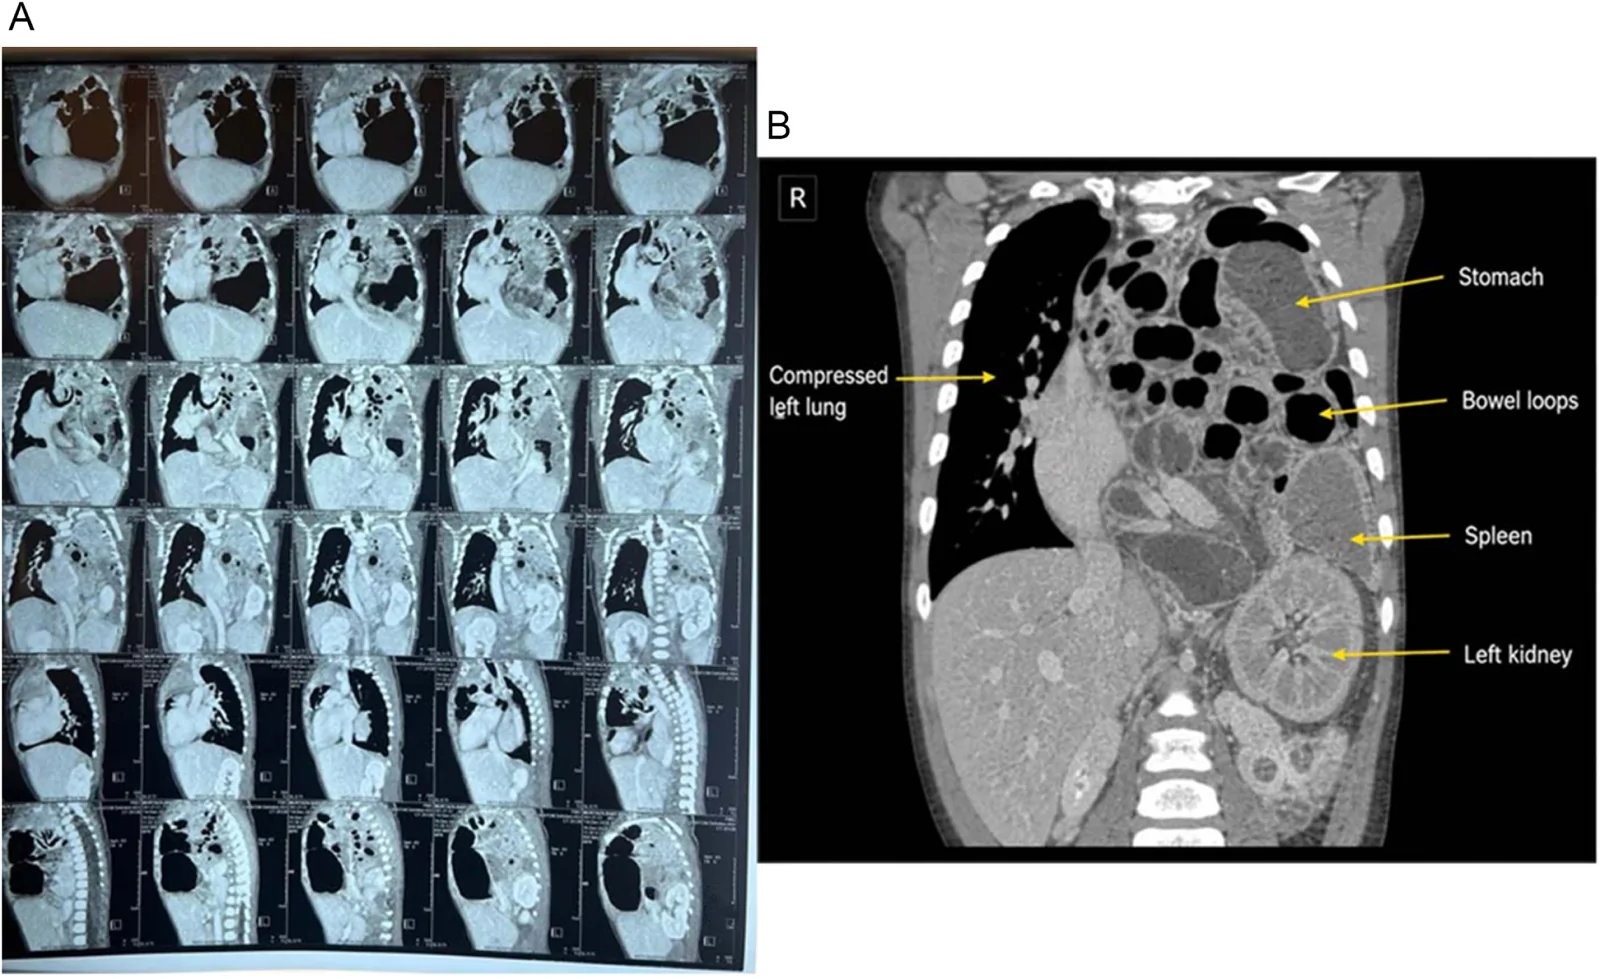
(A) Computed tomography (CT) of the thorax and abdomen demonstrates an extensive left-sided Bochdalek hernia. Multiple abdominal organs, including the stomach, spleen, kidney, and bowel loops, are herniated into the left hemithorax. The displaced viscera cause marked compression of the ipsilateral lung and a mediastinal shift to the right. (B) Coronal CT image shows a left-sided diaphragmatic hernia with herniation of the stomach and bowel loops into the thoracic cavity, resulting in marked compression of the left lung. The spleen and left kidney are identified for anatomical reference.
